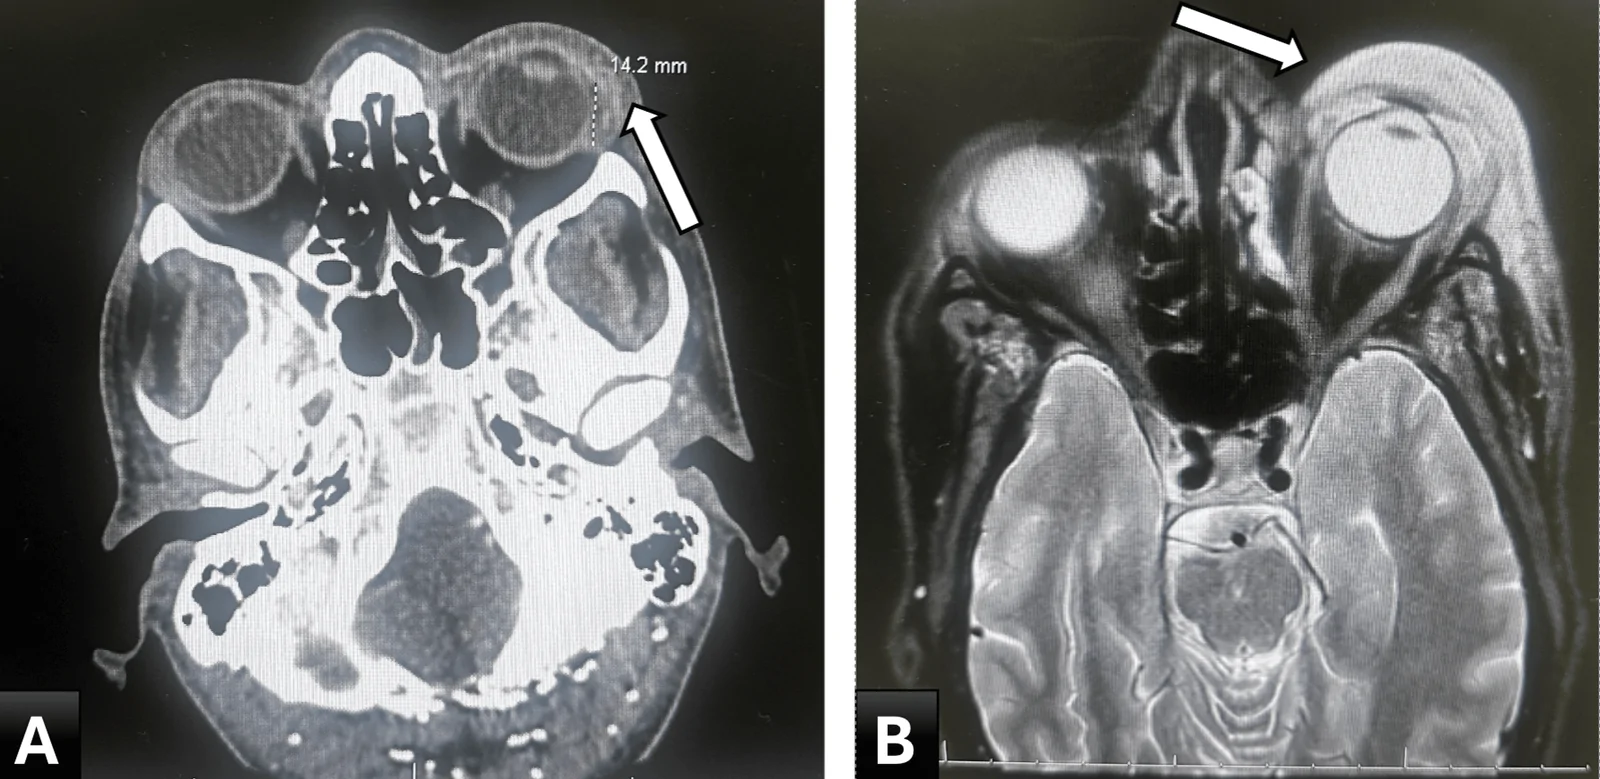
Cross-sectional imaging demonstrating persistent left superior orbital soft tissue thickening (A) Follow-up contrast-enhanced computed tomography (CT) of the orbits demonstrates persistent superolateral left preseptal and periorbital soft tissue thickening (white arrow), measuring up to 14.2 mm, with stable mild proptosis and associated reactive enlargement of the lacrimal gland despite prolonged intravenous antimicrobial therapy. The lesion remained confined to the extraconal compartment without orbital osseous destruction or intraconal extension. (B) Follow-up magnetic resonance imaging (MRI) of the orbits demonstrates persistent abnormal enhancing soft tissue involving the superior left preseptal orbit and palpebral portion of the lacrimal gland (white arrow). The lesion abuts the globe but demonstrates no retrobulbar mass, optic nerve involvement, cavernous sinus extension, or intracranial spread. The persistence of the lesion despite antimicrobial therapy prompted tissue biopsy, which confirmed invasive moderately differentiated conjunctival squamous cell carcinoma.
